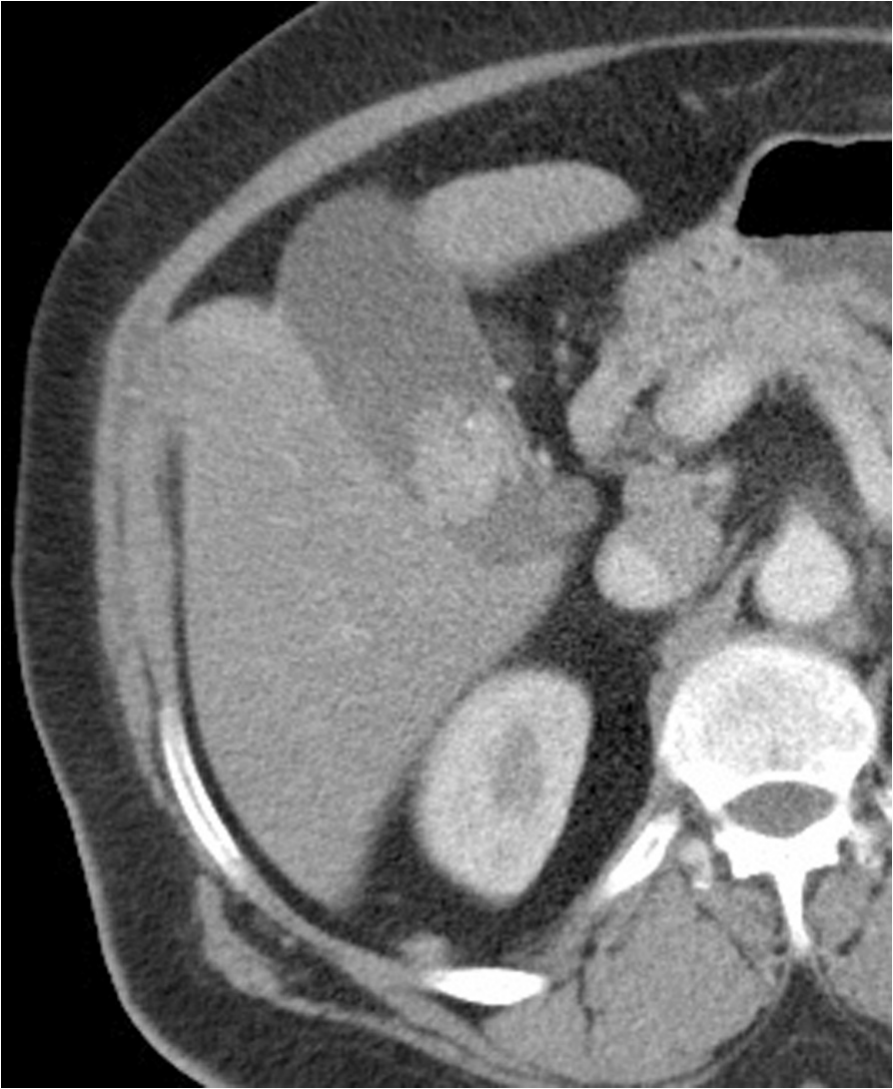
Axial computed tomography image after intravenous contrast enhancement reveals an intraluminal pedunculated hyperdense enhancing mass in the gallbladder without thickening of the gallbladder wall.
